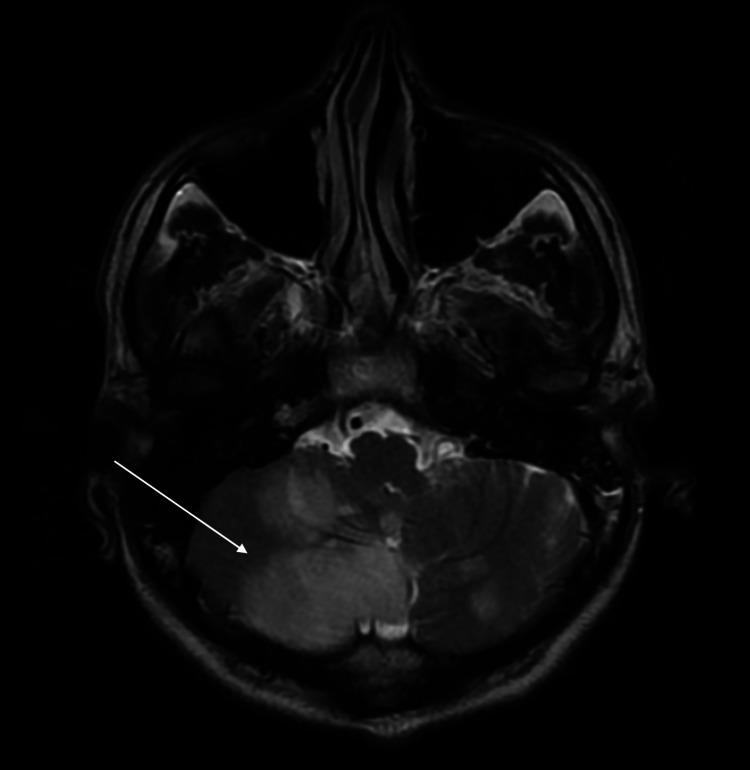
T2/FLAIR MRI shows hyperintensity (arrow) in the right cerebellar hemisphere and few foci in the left cerebellar hemisphere
